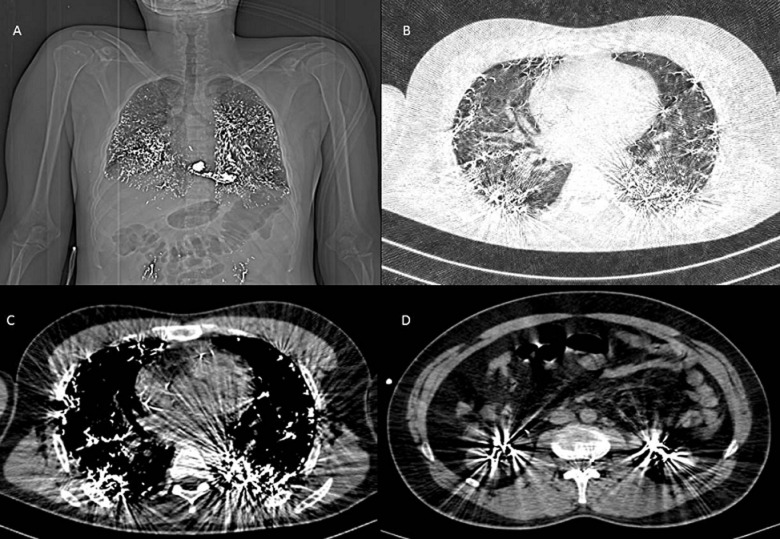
A) bilateral opacities of the patient; B) CT thorax showing high attenuation foci with ground glass opacities in bilateral lung fields; C) CT thorax mediastinal window showing a peripheral distribution with high attenuation focus within right atrium and right ventricle; D) CT abdomen showing high attenuation focus in both kidneys
